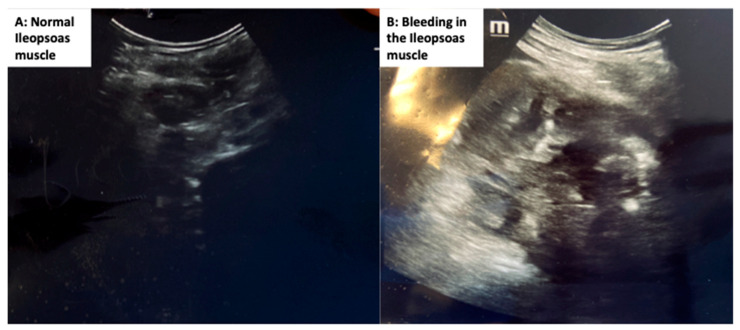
Ultrasound showing iliopsoas muscle bleeding in a patient from our institution.
